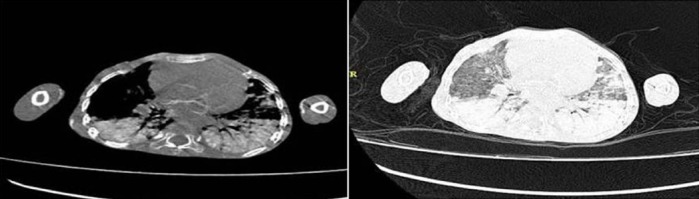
Chest CT showed bilateral hyperdense centrilobular nodules with ill-defined margin suggestive of metastatic calcification throughout both lungs
